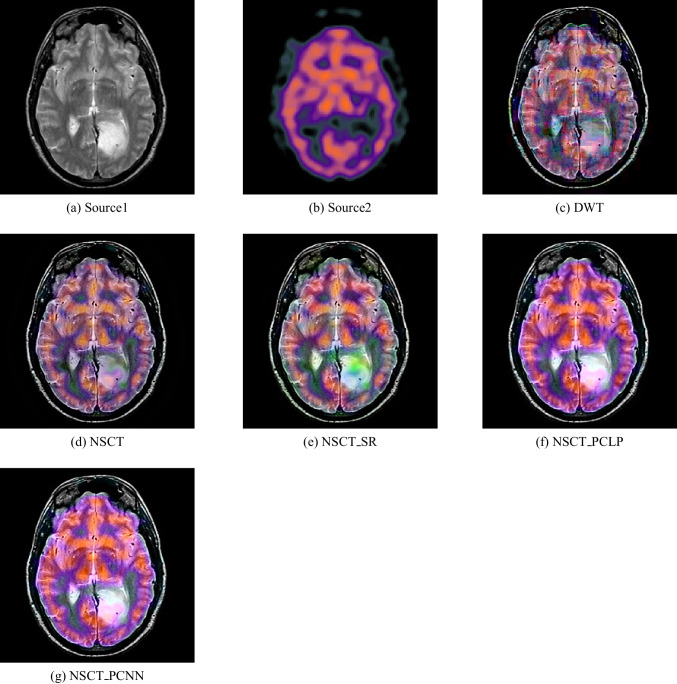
Fusion result of (a) CT and (b) MR-T2 using (c) DWT method, (d) m-PCNN method, (e) CSR method, (f) NSCT method, (g) CSMCA method, (h) GFS method, (i) NSCT-SR method, (j) NSCT-PCLP method, and the proposed method (k) NSCT-PCNN
